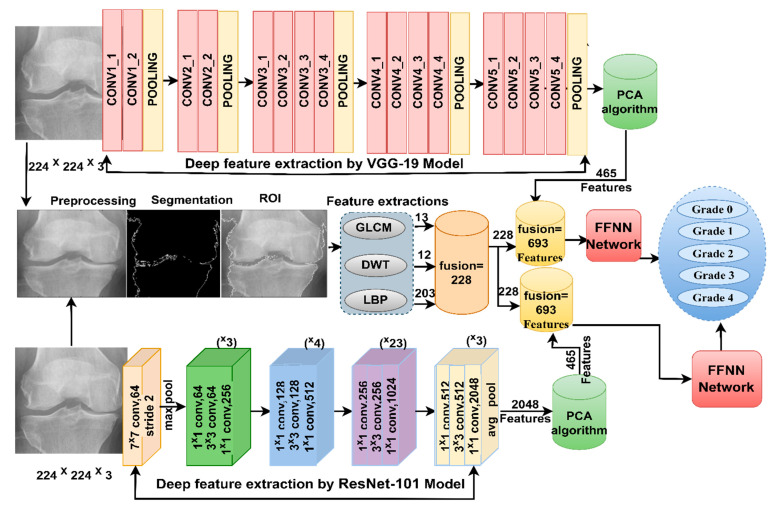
Approaches of the X-ray analysis of the two OAI and RCU datasets for diagnosing osteoarthritis of the knee and discrimination of severity grade using FFNN with fusion features.
